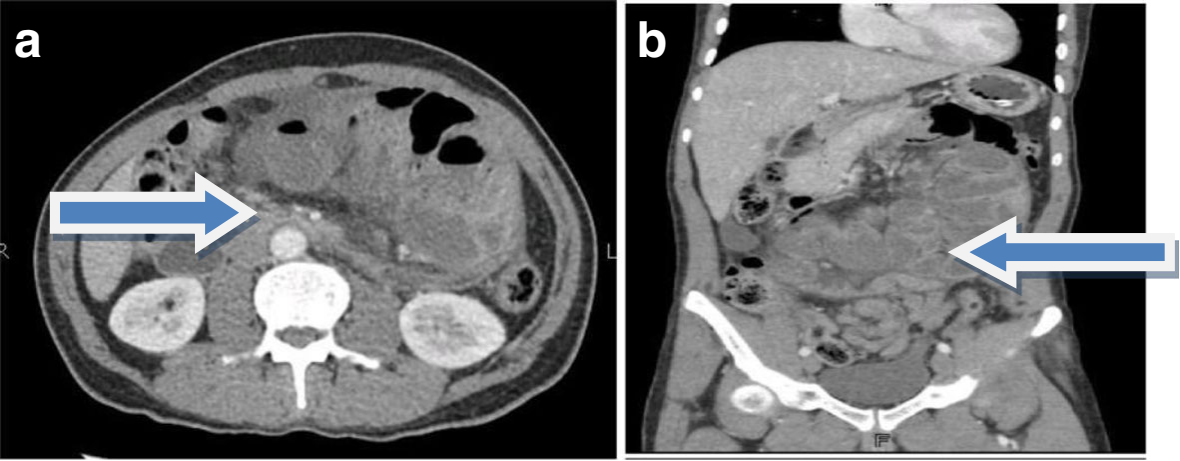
a, b Axial and coronal views of contrast-enhanced computed tomography demonstrates cocooning of abdomen shown by arrows
